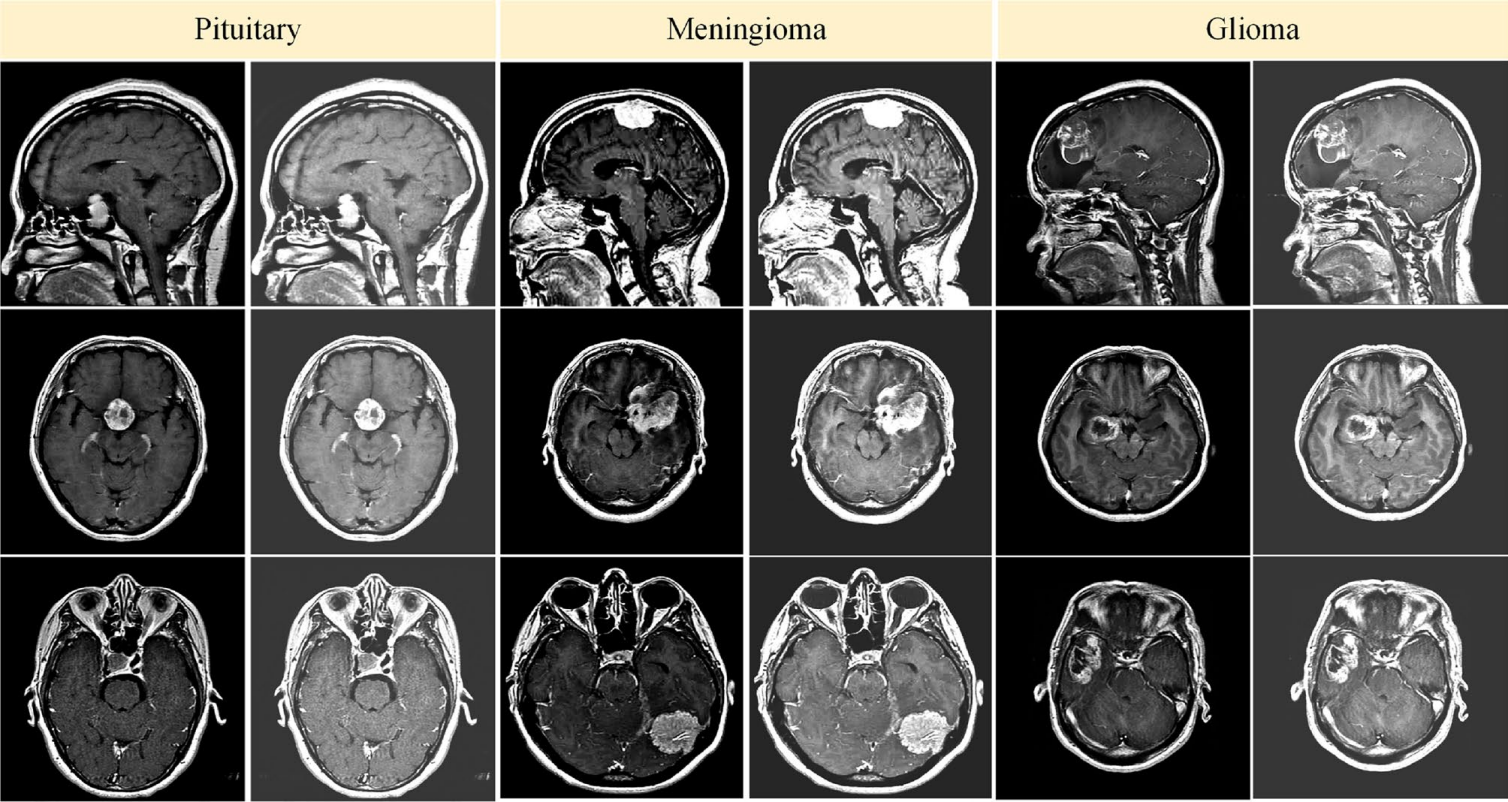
MRI images prior to and following the enhancement operation.
